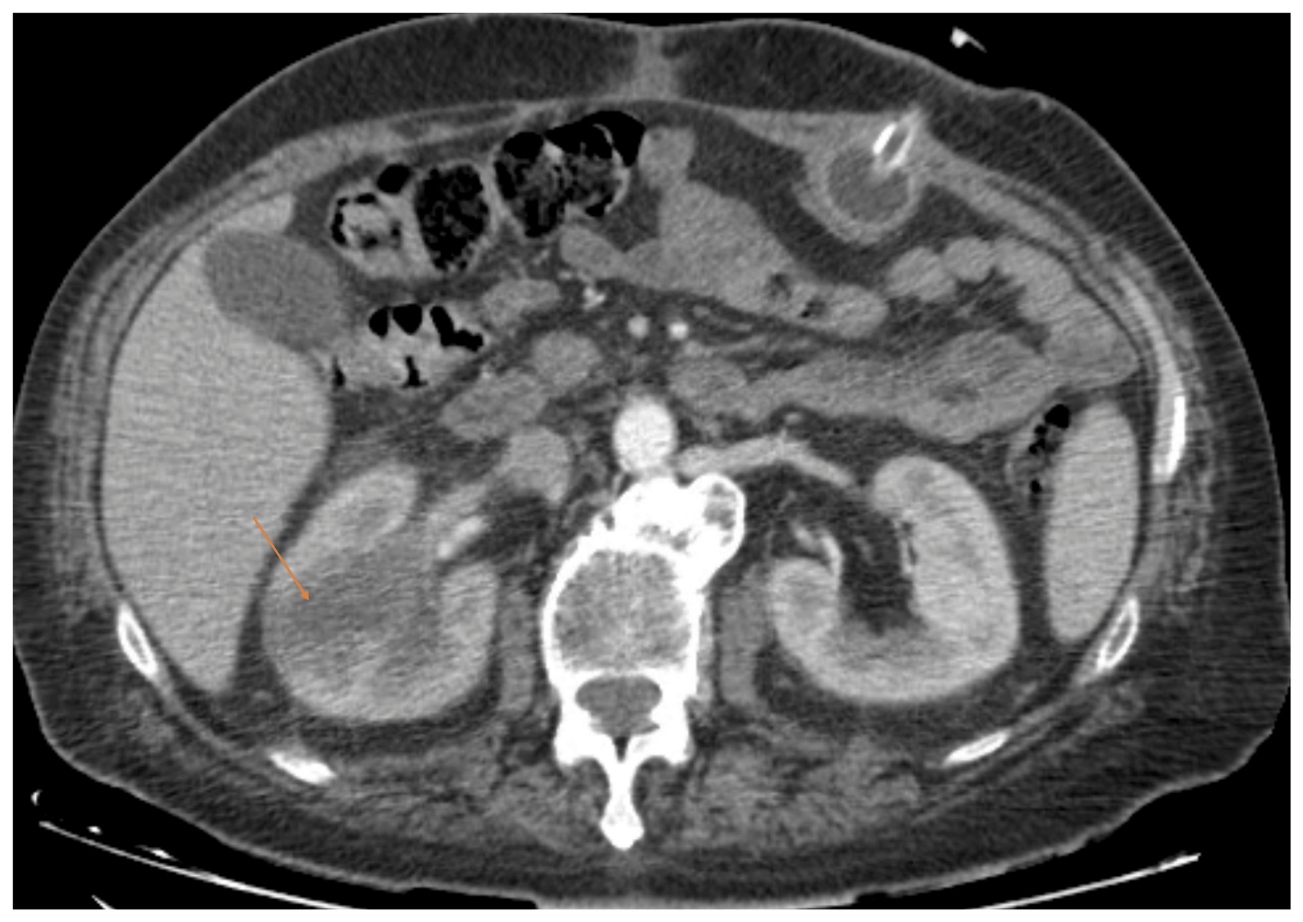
Contrast-enhanced CT of the abdomen showing the right kidney with hypodense lesions (orange arrow) consistent with multifocal pyelonephritis
